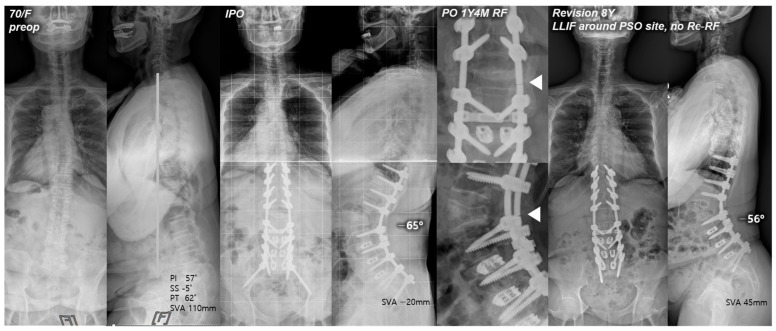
Pre- and postoperative standing radiographs of a 70-year-old female patient. After T10-S1 posterior instrumentation with PSO on L2, and PLIF on L3-S1, optimal sagittal balance was achieved (SVA, −20 mm; TK, 12°; LL, −65°; PI, 57°; PT, 17°; SS, 40°). At 1 year and 4 months after primary deformity correction, RF (right rod) occurred at L2–3. At 8 years following revision surgery with bilateral rod replacement and LLIF around the PSO site, sagittal alignment was well maintained without re-RF. White triangles indicate the site of RF.
